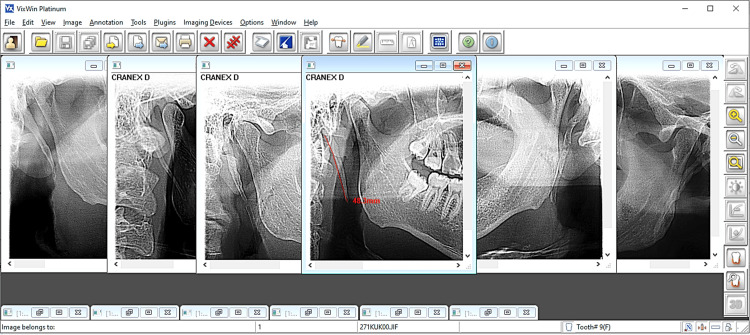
The imaging software used to calibrate, view, and interpret panoramic radiographs, and to measure the styloid process length in this study. Examples of styloid process elongation from the study sample are shown.
